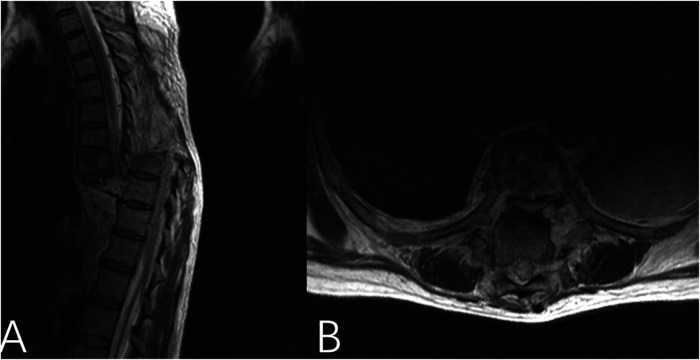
Magnetic resonance imaging (MRI) sequences (A,B) demonstrate complete discontinuity of the spinal column at the T8–T9 segment. Transection of the thecal sac and spinal cord is observed at this level, with interruption of the cerebrospinal fluid (CSF) signal. The proximal and distal spinal cord stumps have retracted, resulting in a conspicuous gap.
